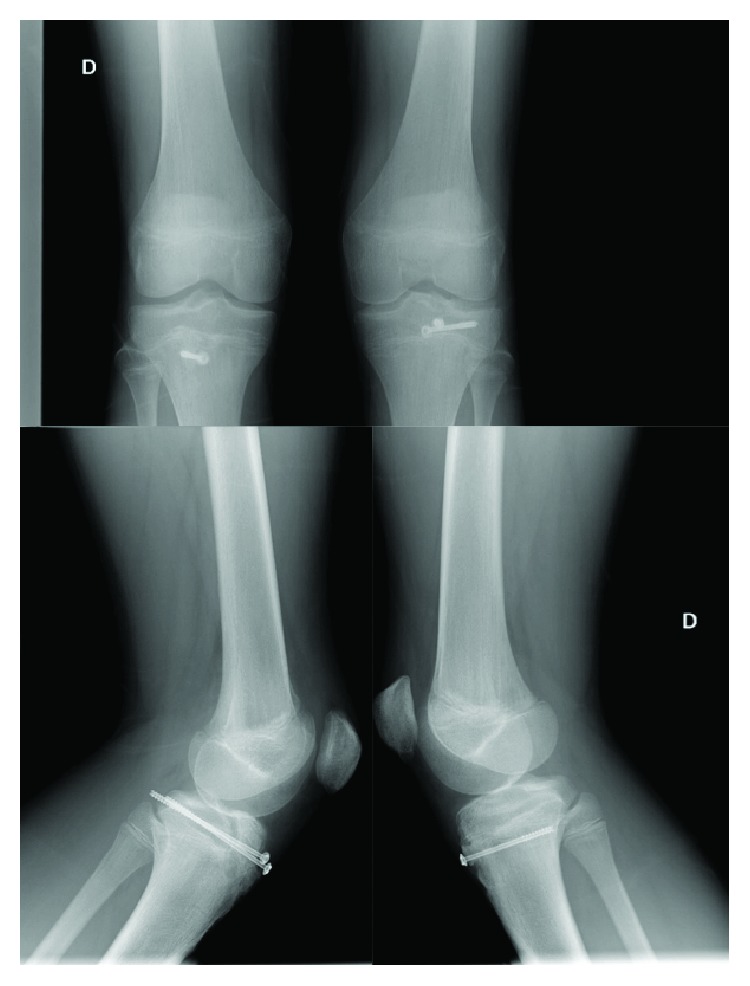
Plain radiographs of both knees at 6 weeks postoperative. Right knee (D) showing one 4.5 mm cannulated screw with a washer and the left one (I) two 4.5 mm cannulated screws with a washer.
